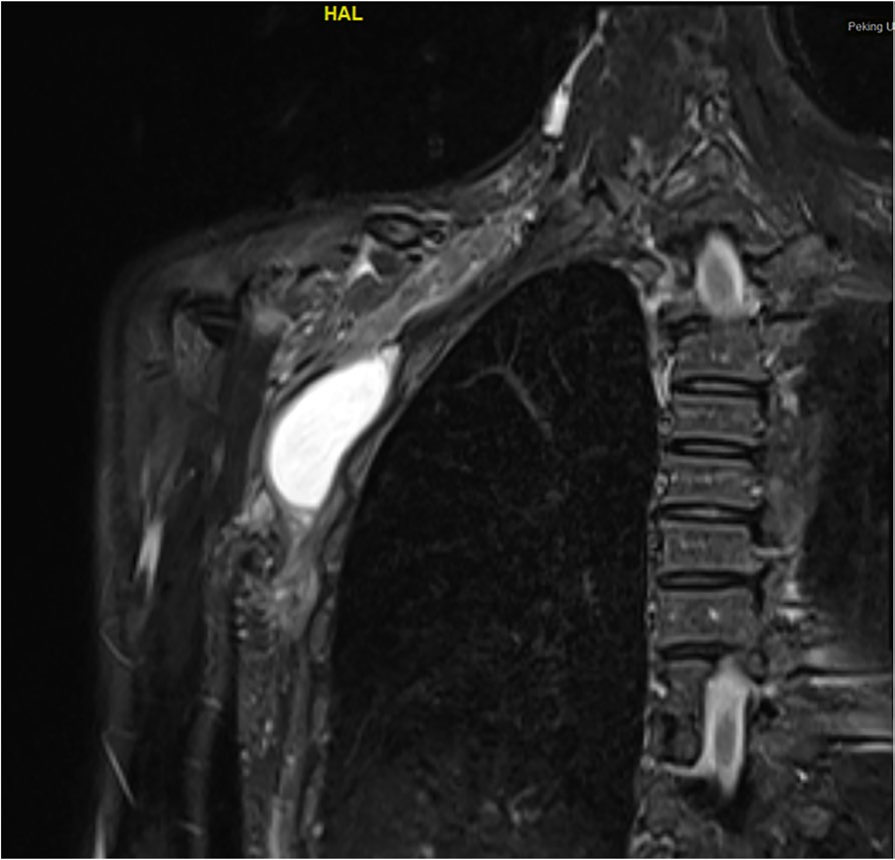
The magnetic resonance imaging manifestation after 3 cycles of irinotecan, vincristine and anlotinib, which induced shrink of the tumor as well as liquefactive necrosis
